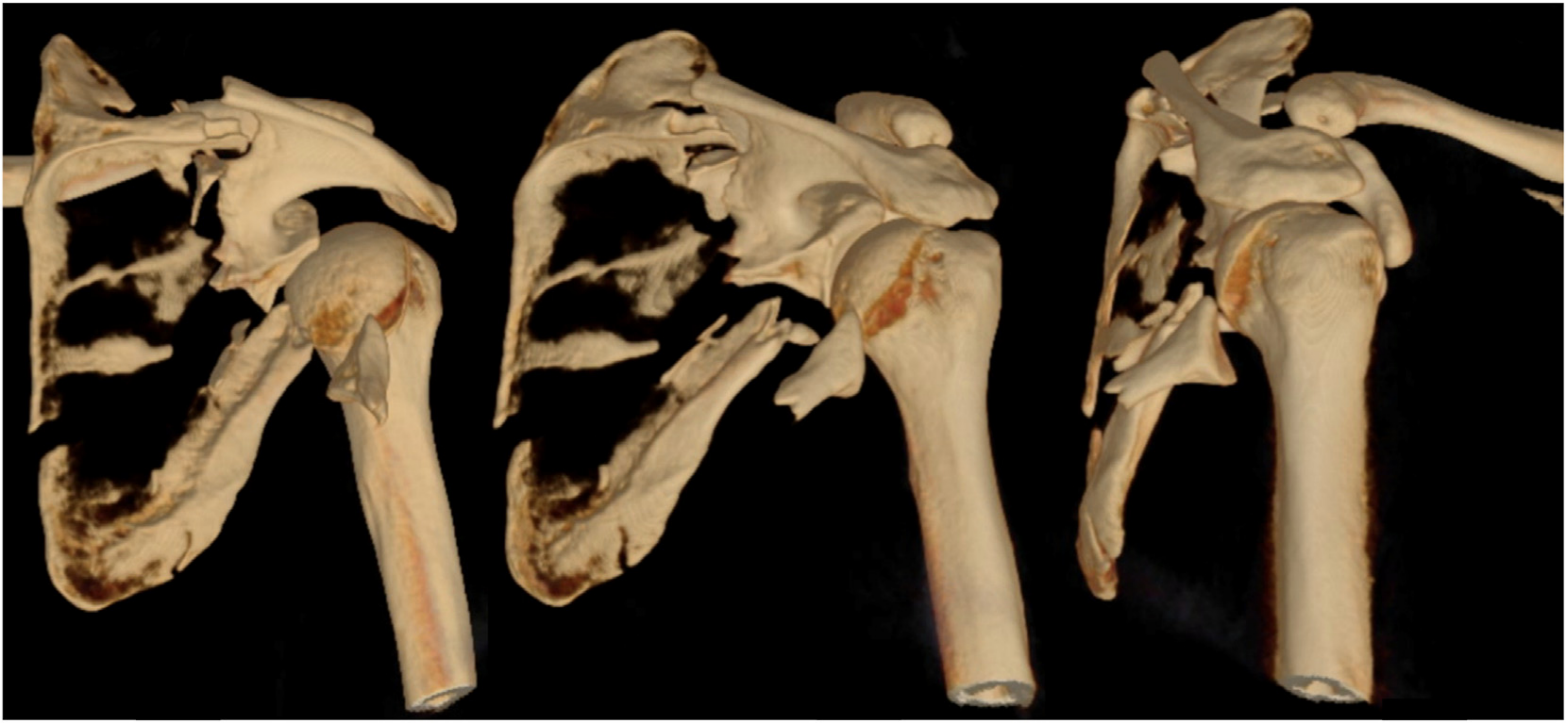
CT scan with 3-dimensional reconstruction. *CT*, computed tomography.
